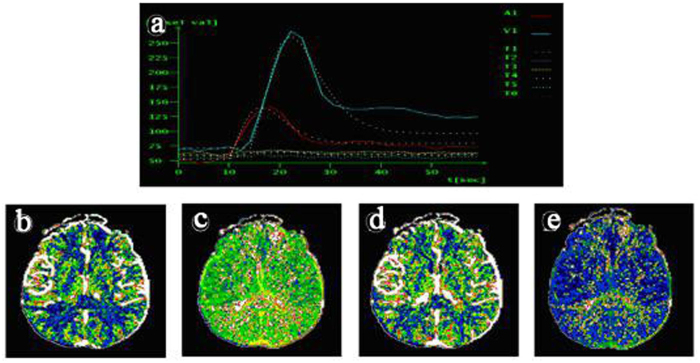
Non-CMS subject, 43-year-old male. Brain CT perfusion images, including (a) time delay curve (TDC) map, (b) cerebral blood flow (CBF), (c) time-to-peak (TTP), (d) cerebral blood volume (CBV), and (e) mean transit time (MTT). On TDC curve, mean peak time for superior sagittal sinus and bilateral MCA arterial perfusion was 22 seconds (blue line) and 18 seconds (red line), respectively.
